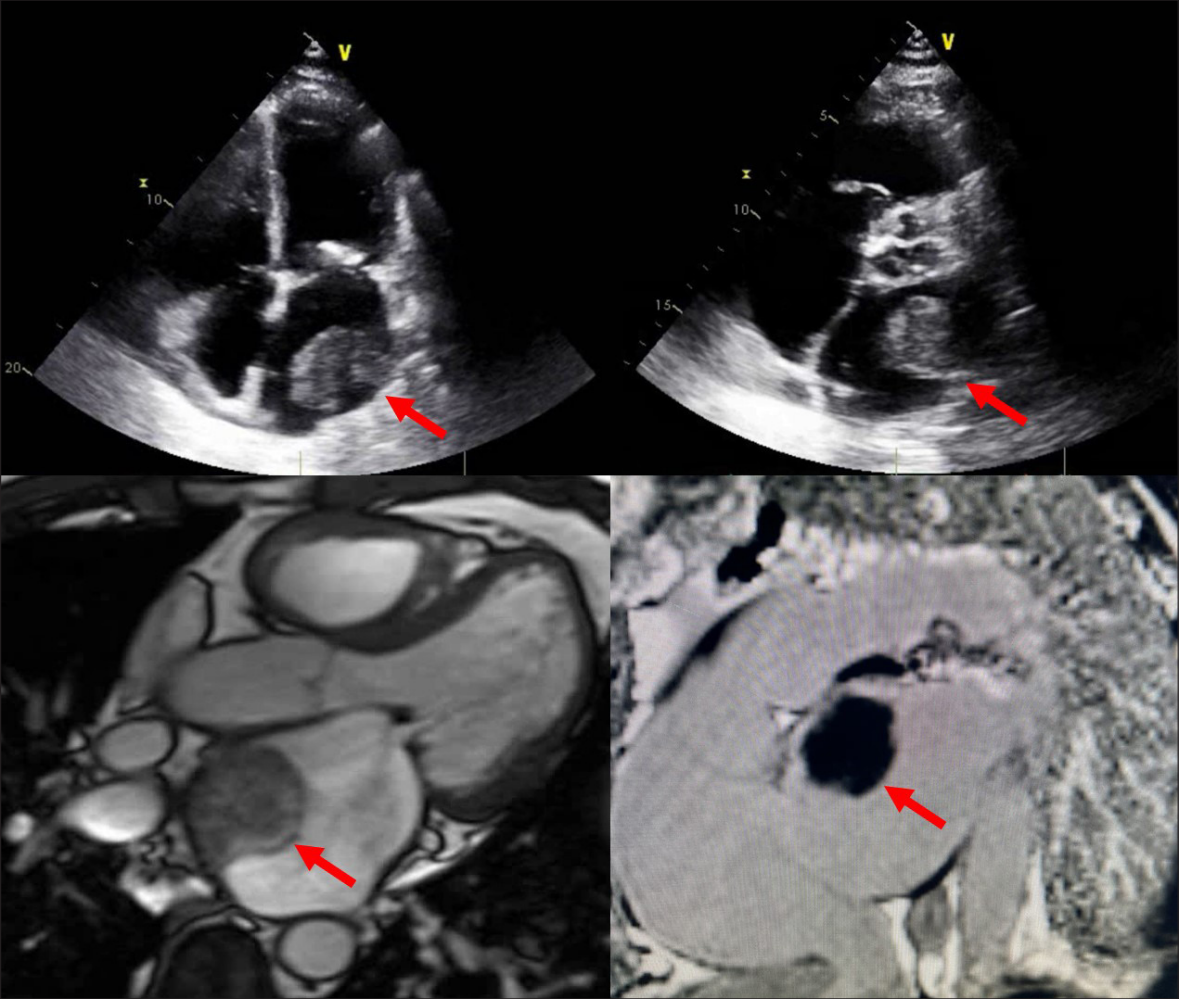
Echocardiography and magnetic resonance imaging showing a thrombus in the left atrial appendage.
